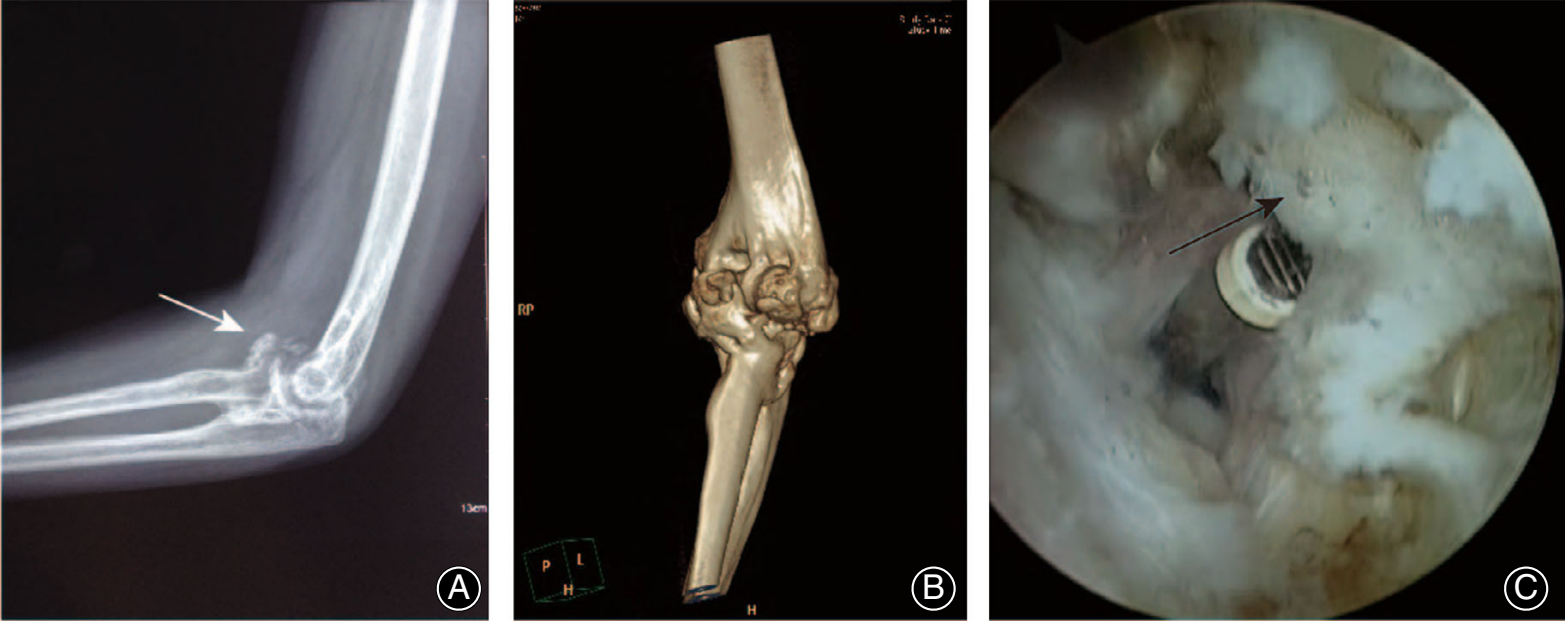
Posttraumatic stiffness with heterotopic ossification (HO) formation in a 56‐year‐old woman. (A, B) Preoperative X‐ray and CT scan shows HO in the front joint. (C) Radiofrequency was used to release the scars around HO.
